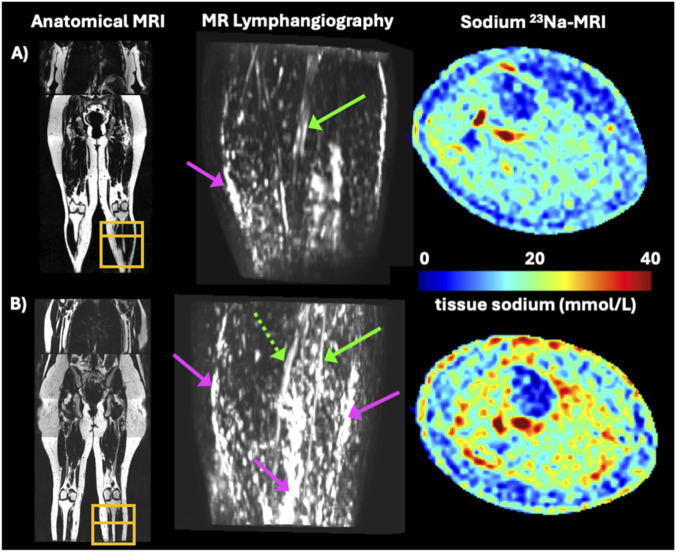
Biomedical imaging by MRI in a case with (A) lipedema (age = 46 years, BMI = 24.9 kg/m^2^) compared to (B) obesity (age = 45 years, BMI = 38.5 kg/m^2^). MR lymphangiography shows features of vascular involvement (green arrows: dilated vessels, dotted arrow: tortuous vessel, pink arrow: tissue edema) to a greater extent in lipedema. Sodium 23Na MRI reveals higher leg tissue sodium in the case of lipedema. Dilated vasculature with excess tissue sodium is interpreted as an inflammatory profile in lipedema which can be imaged *in vivo* with MRI.
